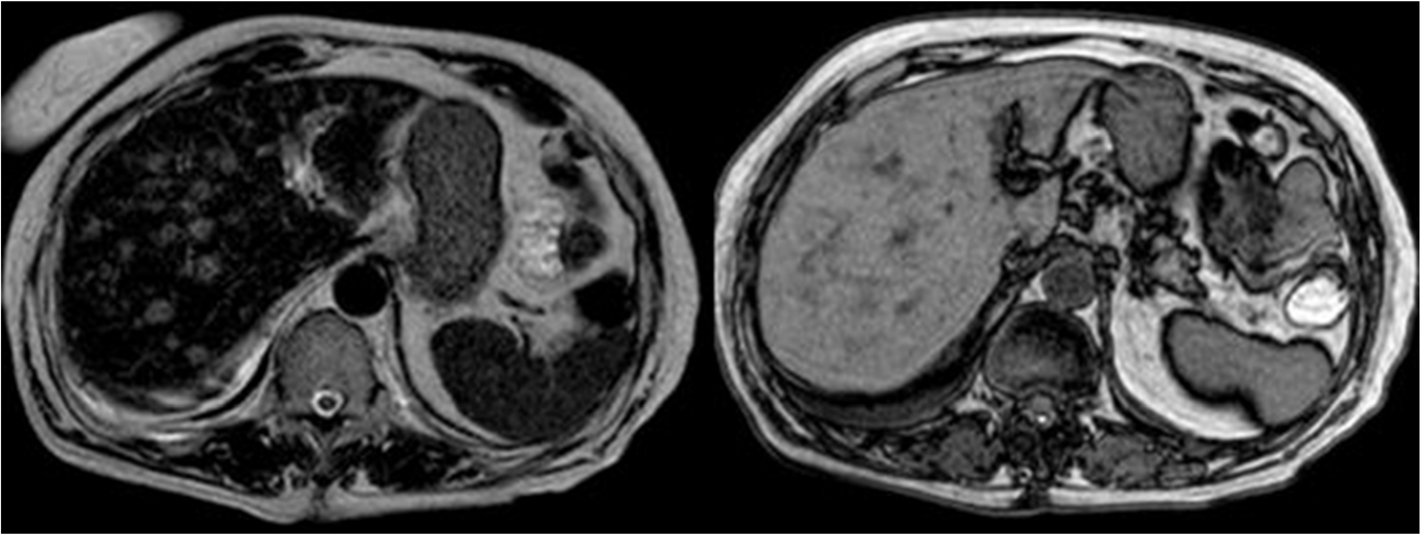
The abdominal MRI confirmed the presence of the lesions, which were hypointense in T1 weighted image and hyperintense in T2 one
